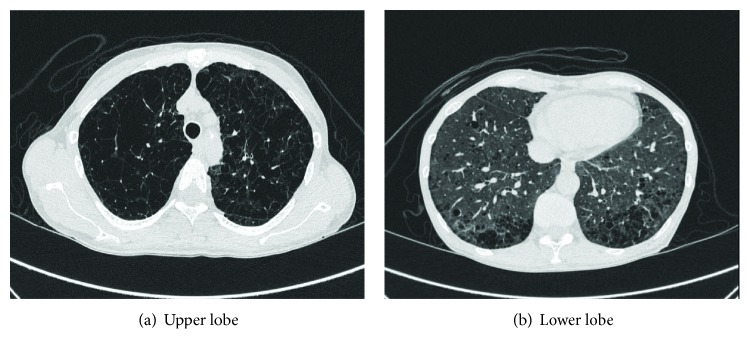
High resolution CT of the chest shows emphysema of the upper lobe (a) and bilateral mosaic pattern of the lower lobe (b).
